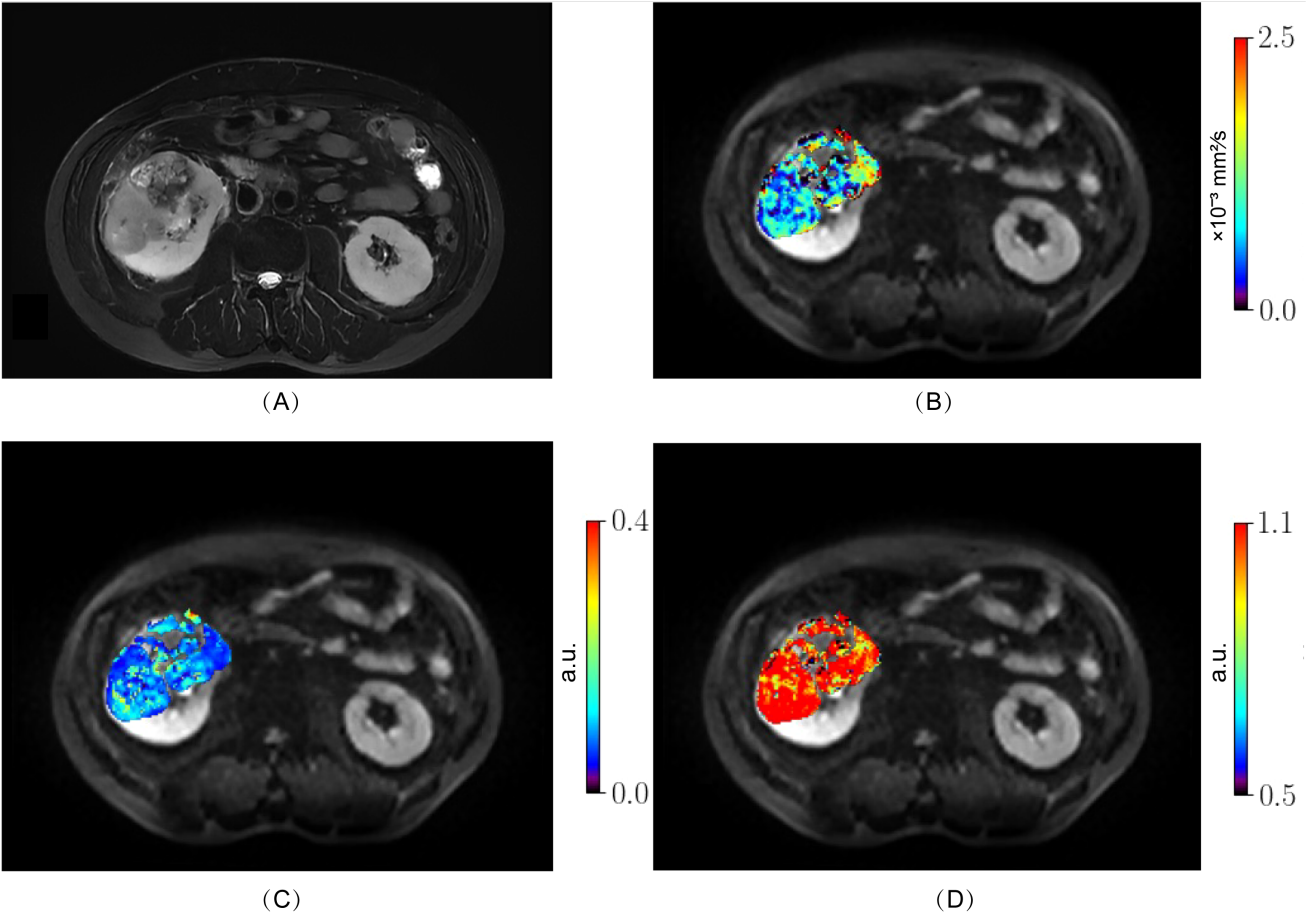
High-grade ccRCC (WHO-ISUP grade 4) in the right kidney of a 50-year-old man. (A), axial fat-suppressed T2-weighted image; (B–D) MR images with quantitative MAD maps. (B), D_h_ values; (C), f_r_ values and (D), α_h_ values. The MAD maps (B–D) show substantial differences between low- (Figure 2) and high-grade tumor (Figure 3). ccRCC, clear cell renal cell carcinoma; MAD, multimodal apparent diffusion model.
